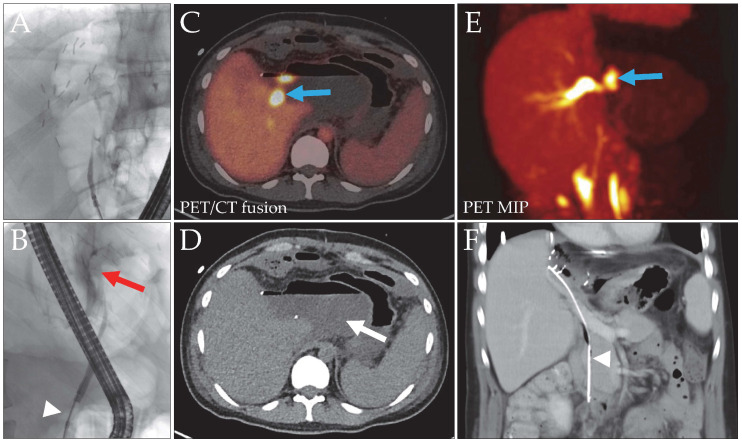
Patient 3, liver donor, with a bile leak after left hemihepatectomy for LDLT. The initial ERC image (A) did not show the biliary tree. With a blocked catheter ((B), white arrowhead), a contrast media extravasation (red arrow) without clear leak localization was evident. Intervention with stent placement was not possible due to the stricture. DAZA-PET (C,E) revealed a central bile leak (blue arrows, SUVmax 43.0/SUVmax/mean liver tissue 9.4/7.0), draining into a large bilioma ((D), arrow). The space-occupying bilioma caused external compression of the central bile ducts, resulting in distention of the right intralobar ducts. After bilioma and leak repair surgery, a long stent was implanted to facilitate bile outflow ((F), arrowhead). MIP, maximum intensity projection.
